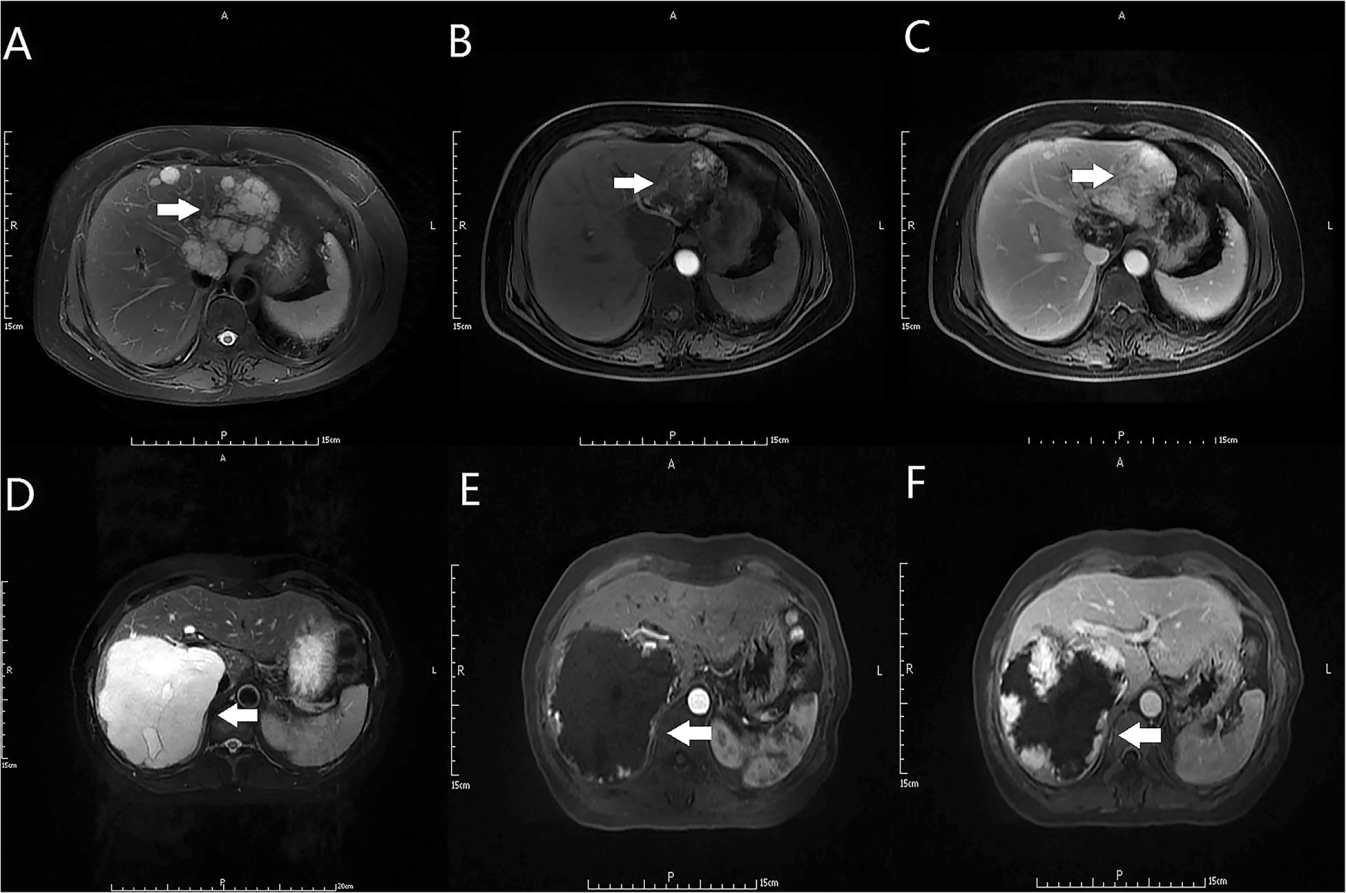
Magnetic resonance imaging of giant liver haemangiomas. a and d T2-weighted magnetic resonance shows giant liver haemangiomas on the left and right liver (A and D, arrow). b and e Magnetic resonance in the arterial phase shows giant liver haemangiomas on the left and right liver (B and E, arrow). c and f Magnetic resonance in the delayed phase shows giant liver haemangiomas on the left and right liver (C and F, arrow)
